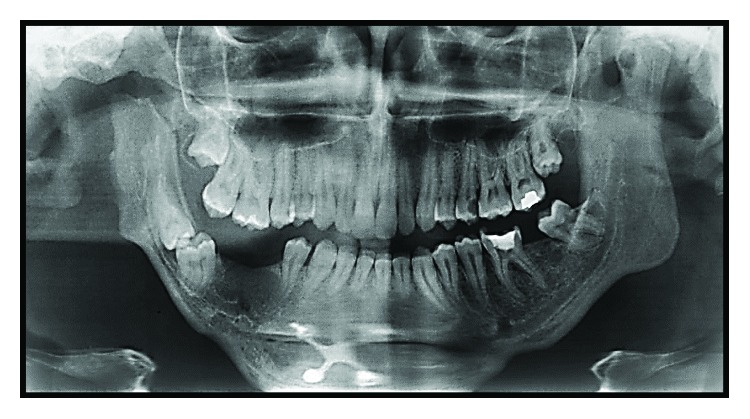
Panoramic radiography after two years emphasizing stability in the chin fixation and with no signs of ankylosis relapse.
